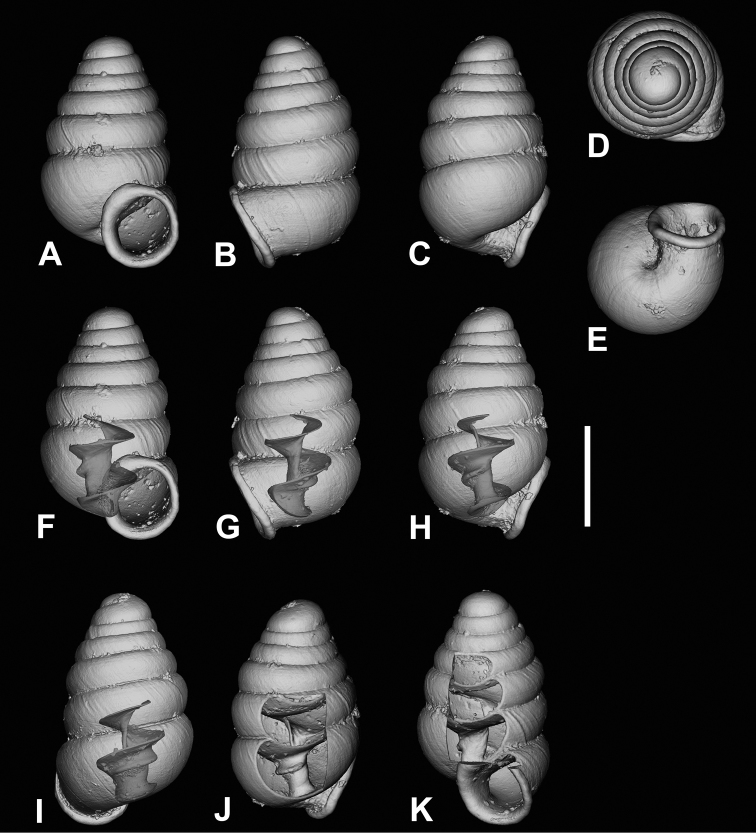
CT images of *Zospeumsuarezi* syn. n. of *Z.schaufussi* von Frauenfeld, 1862, paratype *Z.suarezi* (Gittenberger 1980), RMNH.MOL.55389 from type locality. Scale bar: 500 µm.
